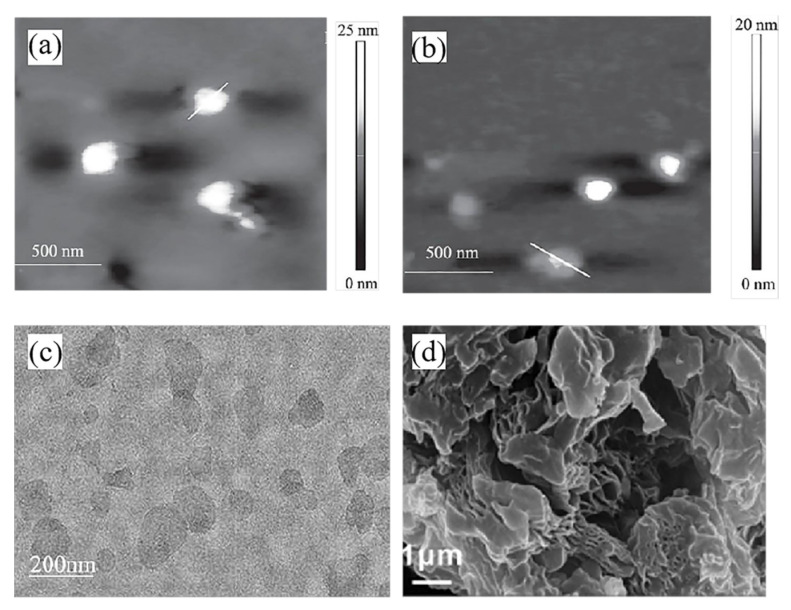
Images of lamellar stripping products of two-dimensional materials. (a) AFM image of exfoliated CTA-Mt [55], (b) AFM image of exfoliated MgAl-SDS-LDH [55], (c) TEM image of stripped Aco-LDH nanosheets [56], (d) SEM image of exfoliated LDH [50].
